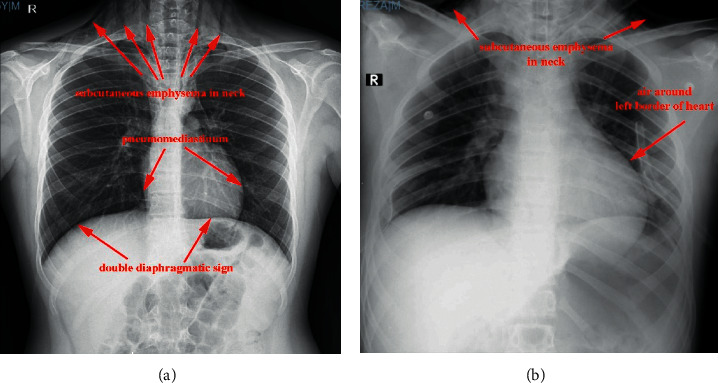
Chest X-ray revealing double diaphragmatic sign, air around the left border of the heart, and subcutaneous emphysema in the neck associated with pneumomediastinum.
